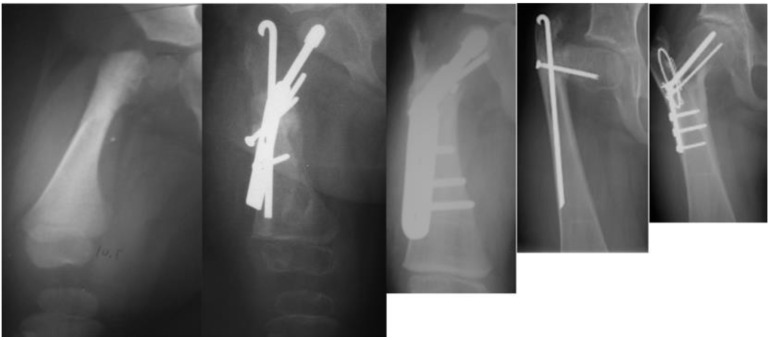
Radiographs showing: preoperative type 1b CFD (leftmost); after SUPERhip procedure fixed with infant sliding hip screw (second to left); the neck did not ossify so the infant sliding hip screw was changed to a larger size sliding hip screw (revision SH) a year later (middle); delayed ossification of the neck persisted and two years later the hip screw was removed and recurrent varus deformity occurred (second to right); a second revision SH was performed with blade plate fixation and insertion of BMP2 and the neck fully ossified (rightmost).
